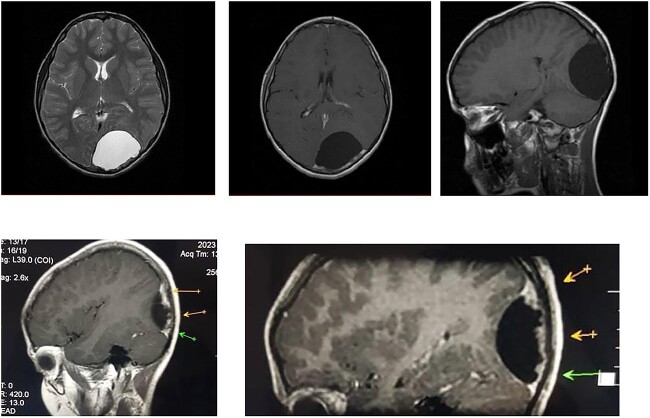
Brain MRI scan of the Hydatid Cyst. Meningeal enhancement around posterior margin of the cyst is seen suggestive for intradural location, the cyst is not exceeding the mid line as well.
